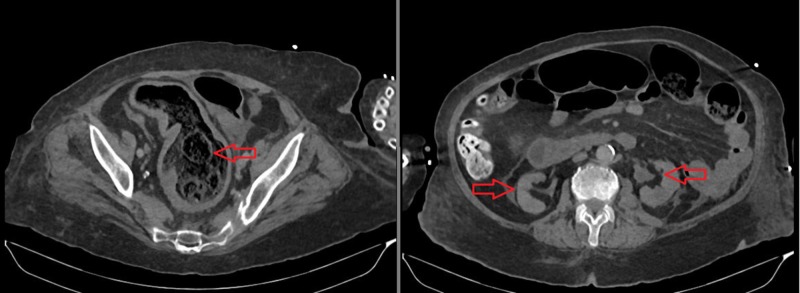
Non-contrast CT taken on day two of the admission After rectosigmoidal lavage, a decrease in the amount of fecaloid in the rectum (left, red arrow) and regression in the grade of hydroureteronephrosis (right, red arrows) can be seen CT: computed tomography
